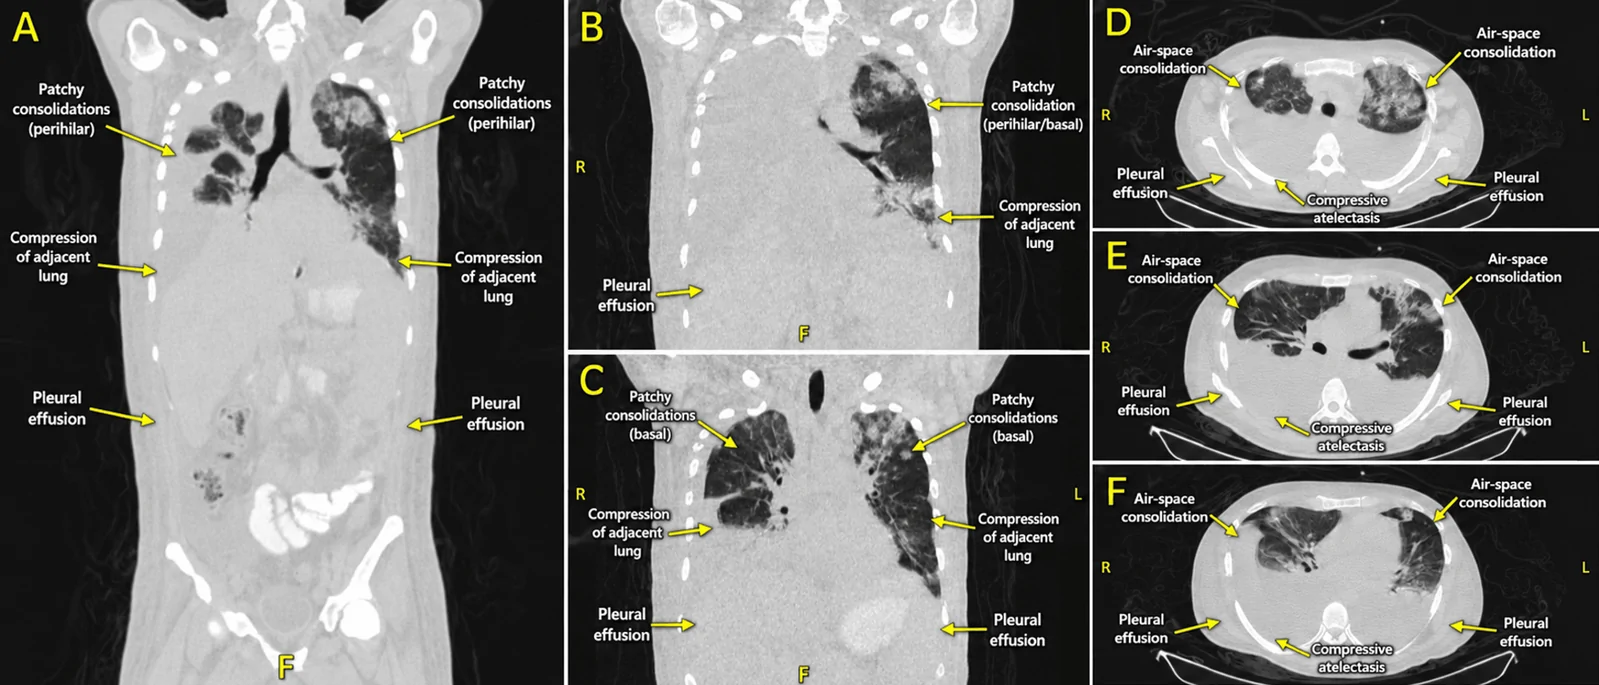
Follow-up computed tomography showing progression of thoracic involvement. Panels A–C: Coronal CT images demonstrate extensive bilateral pulmonary involvement characterized by patchy consolidations with a predominantly perihilar and basal distribution. There is evidence of associated bilateral pleural effusion occupying a significant portion of the thoracic cavity, contributing to partial compression of the underlying lung parenchyma. Additionally, findings suggest increased soft tissue density consistent with generalized lymphadenopathy and possible associated systemic involvement. Panels D–F: Axial CT images (lung window) confirm the presence of bilateral pleural effusions with compressive atelectasis of adjacent lung segments. Patchy areas of consolidation and interstitial involvement are observed, without well-defined cavitary lesions. The distribution and extent of findings are suggestive of a progressive systemic process rather than a localized pulmonary infection. Overall, the imaging findings demonstrate clear radiological progression compared to prior studies, with development of pleural effusions, worsening parenchymal involvement, and features consistent with systemic disease, correlating with the patient’s clinical deterioration.
